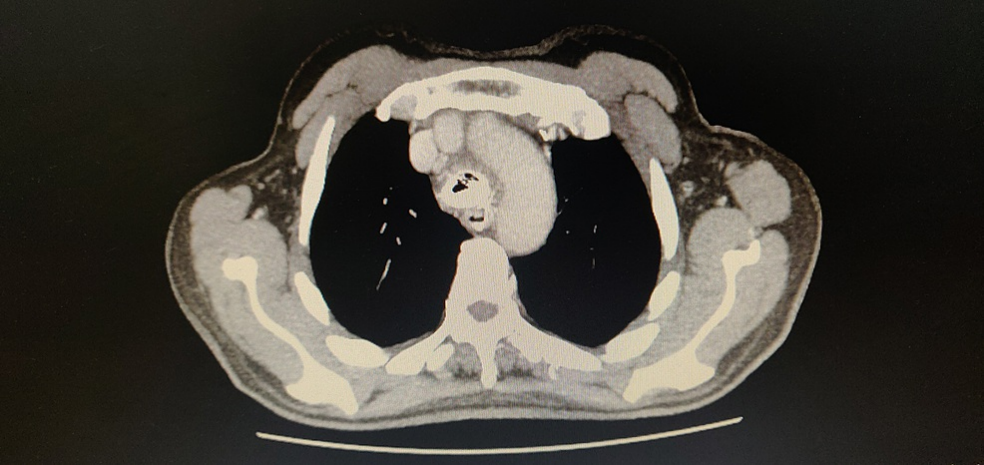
Preoperative CT chest showing fistulous communication between trachea and esophagus caused by tracheal stent - image 1 CT: computed tomography
